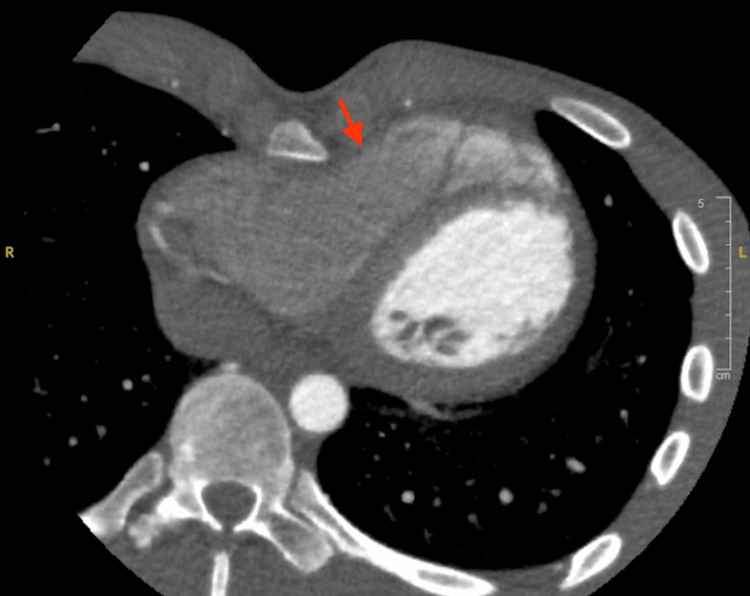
Axial chest CT demonstrating severe pectus excavatum with an arrow highlighting focal right ventricular compression.
